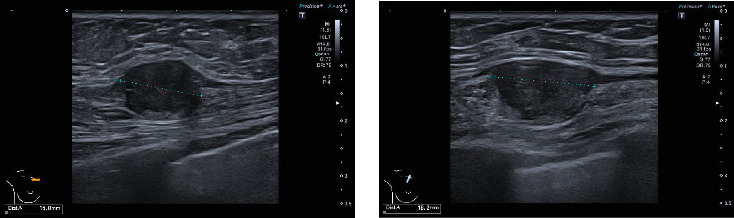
Breast ultrasound images demonstrating the original 15 mm × 18 mm mass on the upper right chest wall area which was originally biopsied (27/04/2023).
